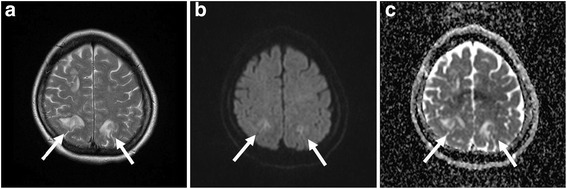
T2-weighted (a) and diffusion-weighted (b) magnetic resonance images 10 hours after presentation show increased signal intensity in both parietooccipital lobes (*arrows*). The lesions also show increased signal intensity on apparent diffusion coefficient map images (*arrows*) (c)
